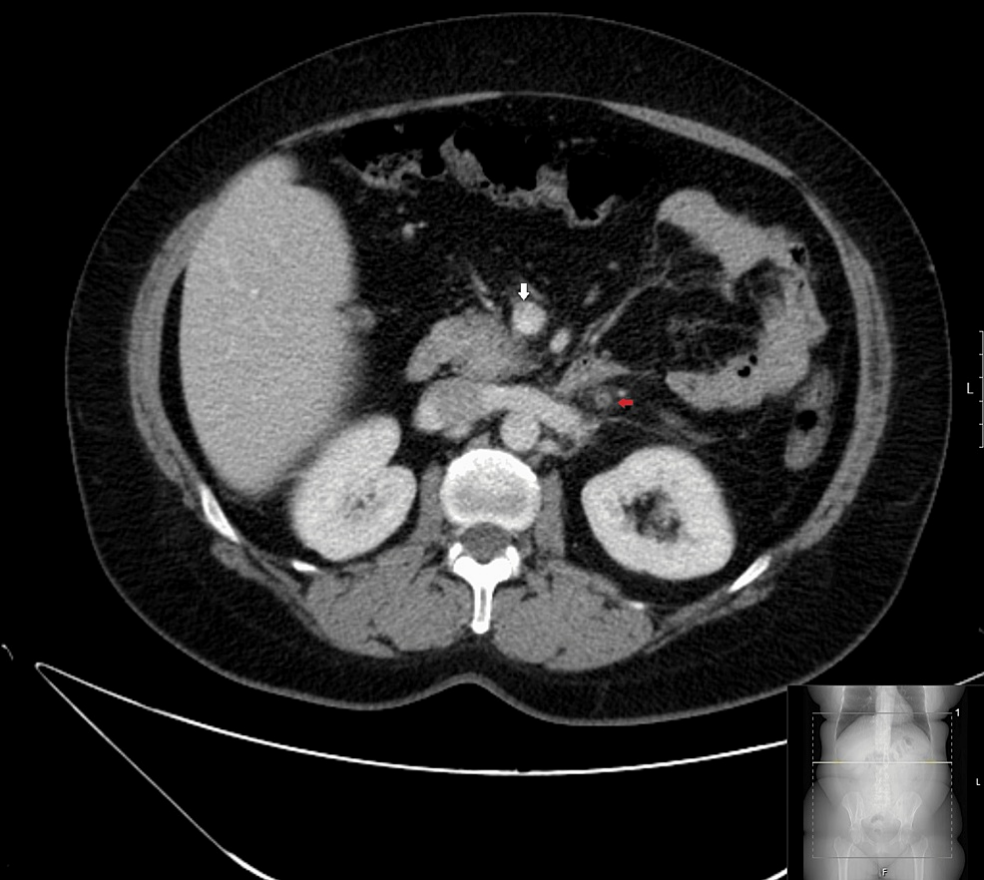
CT with axial reformatting after intravenous contrast administration (portal phase). The thrombosed inferior mesenteric vein is identified, without endoluminal contrast (red arrow). Note the normal contrast filling of the superior mesenteric vein (white arrow). CT: computed tomography
